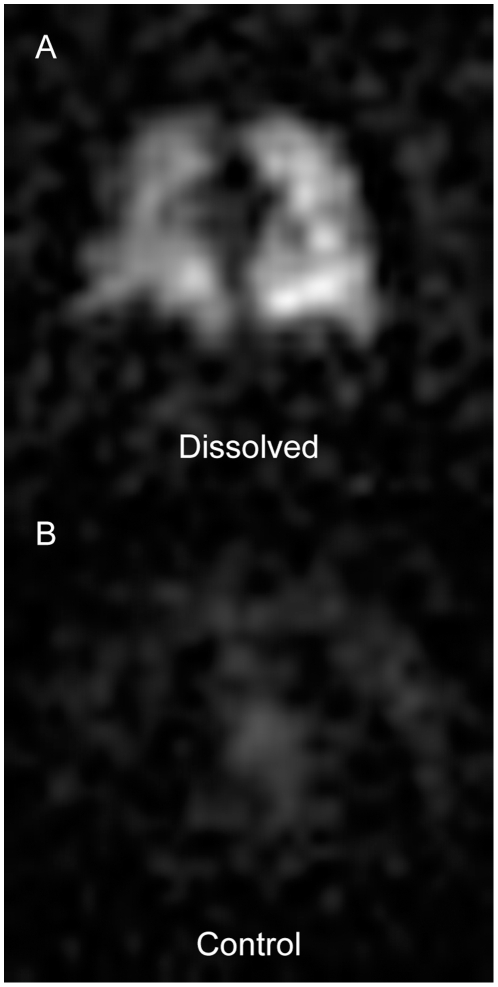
Off-resonant excitation of gas-phase ^129^Xe magnetization. (A) Representative 15-mm-thick section from a standard dissolved HP ^129^Xe image (α = 8°, RF centered 3826 Hz above the gas-phase resonance) of a supine subject. (B) Corresponding 15-mm-thick section from a control image of the same supine subject. The MRI acquisition parameters were identical to those used to produce the image in (A) except that the RF pulse was centered 3826 Hz below the gas-phase resonance. Windowing and leveling were identical for both (A) and (B).
